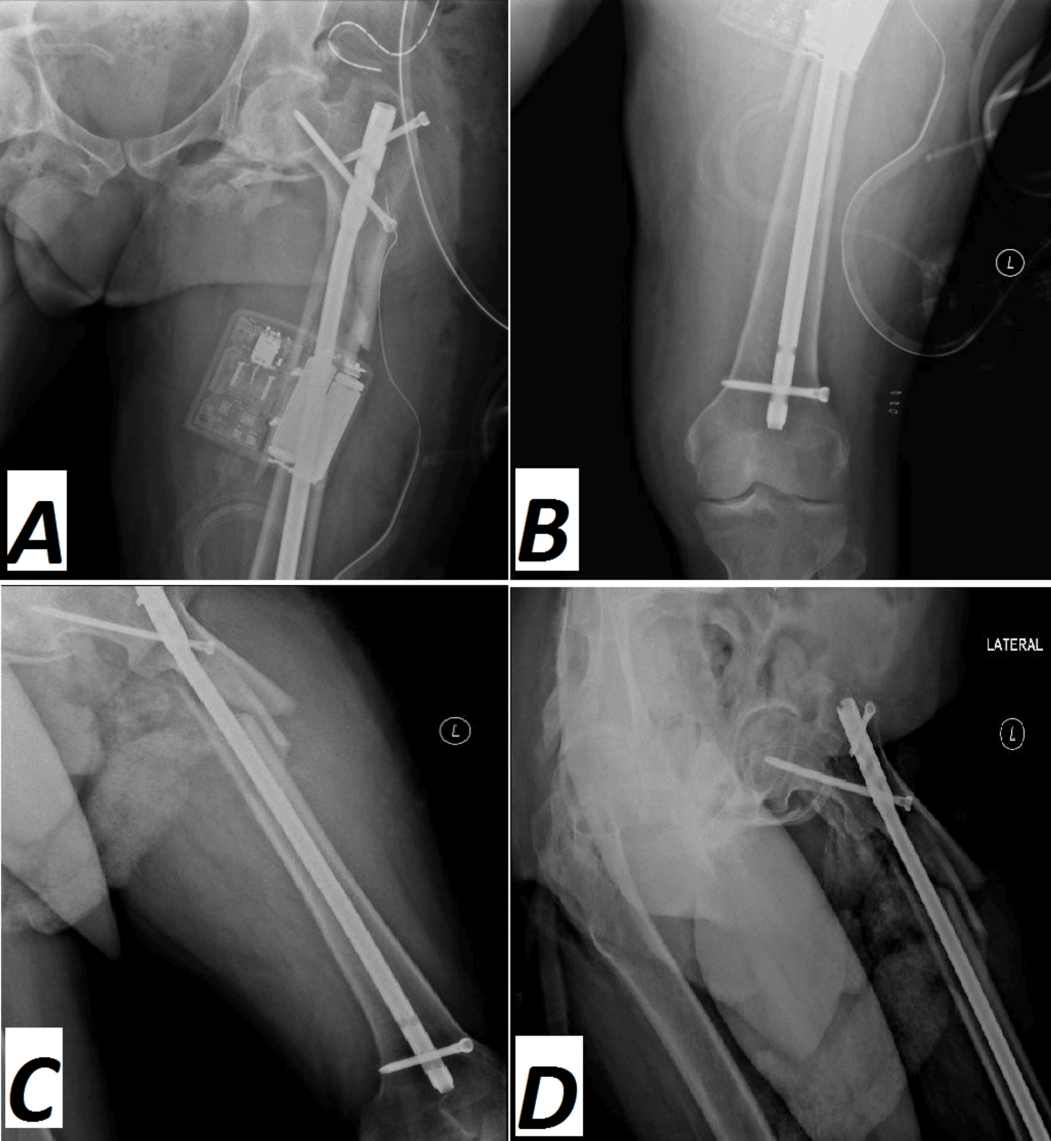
Immediate postoperative anteroposterior and lateral radiographs (A, B, C, D) of the left femur show a reduced, severely comminuted subtrochanteric fracture, fixed by an antegrade intramedullary nail.
